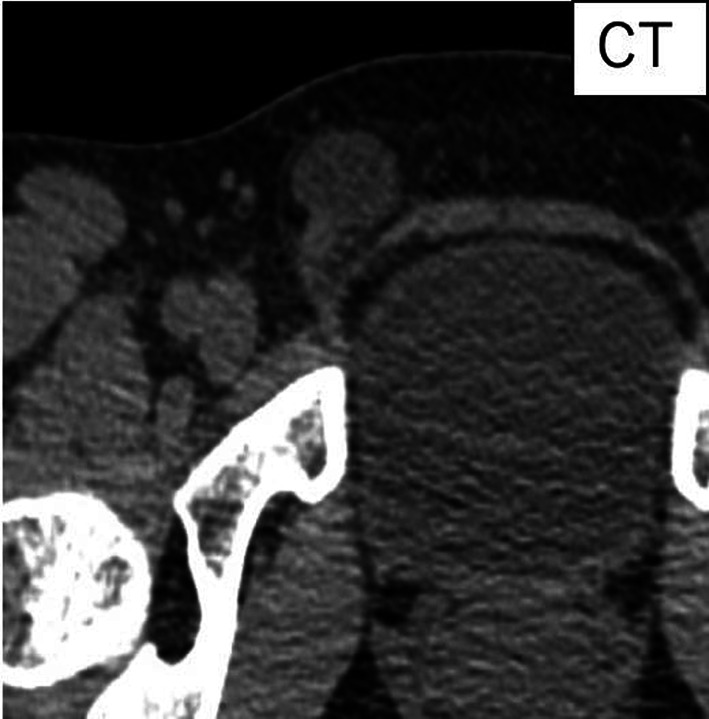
Abdominal computed tomography revealed a 20 × 20 mm cystic mass in the right inguinal region
